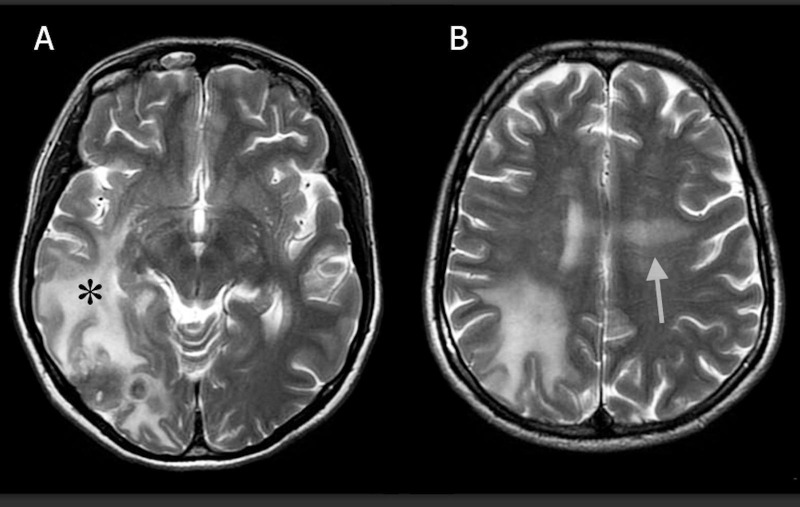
Magnetic resonance imaging T-2 weighted image (MRI T2WI) (A) MRI T2WI axial sequence demonstrates extensive perilesional edema extending anteriorly and inferiorly into the right temporal lobe indicated by the black asterisk. (B) MRI T2WI image demonstrates bifrontal subcortical edema extending across the body of the corpus callosum indicated by the white arrow.
